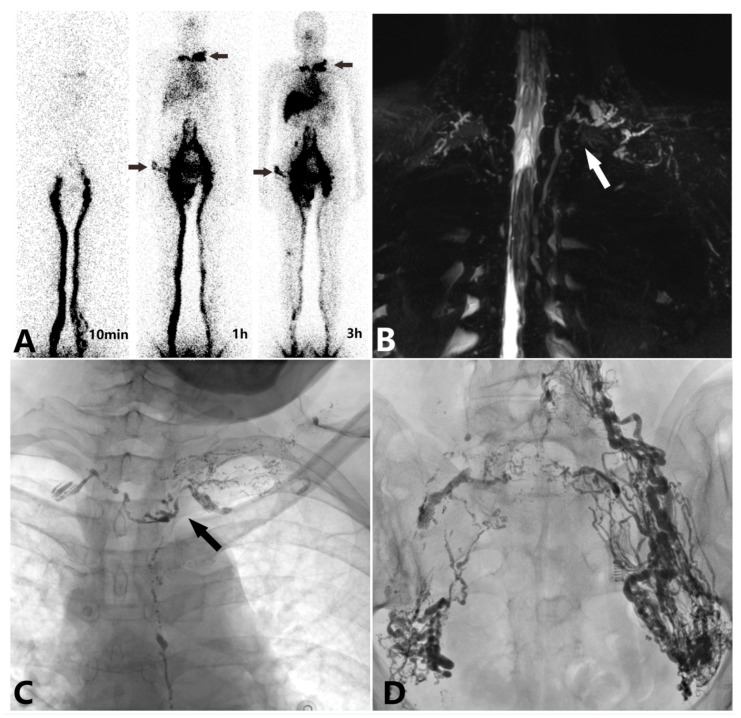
A 62-year-old female with a 6-month history of genital edema and chyle leak. (A) ^99^Tc^m^-DX lymphoscintigraphy demonstrated increased radioactive accumulation in the pelvic and perineal regions, along with abnormal accumulation at the subclavian–jugular venous angle (black arrow). (B) Non-enhanced MRL images of the thoracic duct (TD) showed the dilatation and structural disruption of the TD at the venous angle, along with the abnormal visualization of collateral lymphatic trunks (white arrow). (C) Intranodal lymphangiography revealed an obstruction and structural abnormalities at the thoracic duct (TD) outlet (black arrow). Lipiodol failed to enter the bloodstream, instead refluxing through the subclavian lymph trunk, jugular lymph trunk, and right lymphatic duct. (D) Intranodal lymphangiography further showed the dilation, reflux, and structural disruption of the pelvic lymphatic vessels, with lipiodol refluxing to the contralateral side (right).
